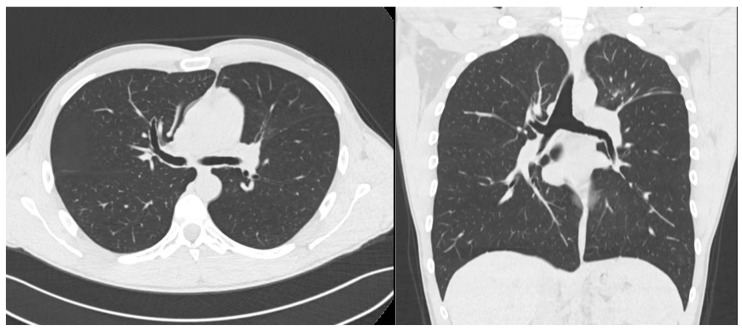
From left to right: Increased left and right main bronchus diameter after endoscopic and systemic treatment (CT scan axial projection). Increased left and right main bronchus diameter after endoscopic and systemic treatment (CT scan coronal projection).
